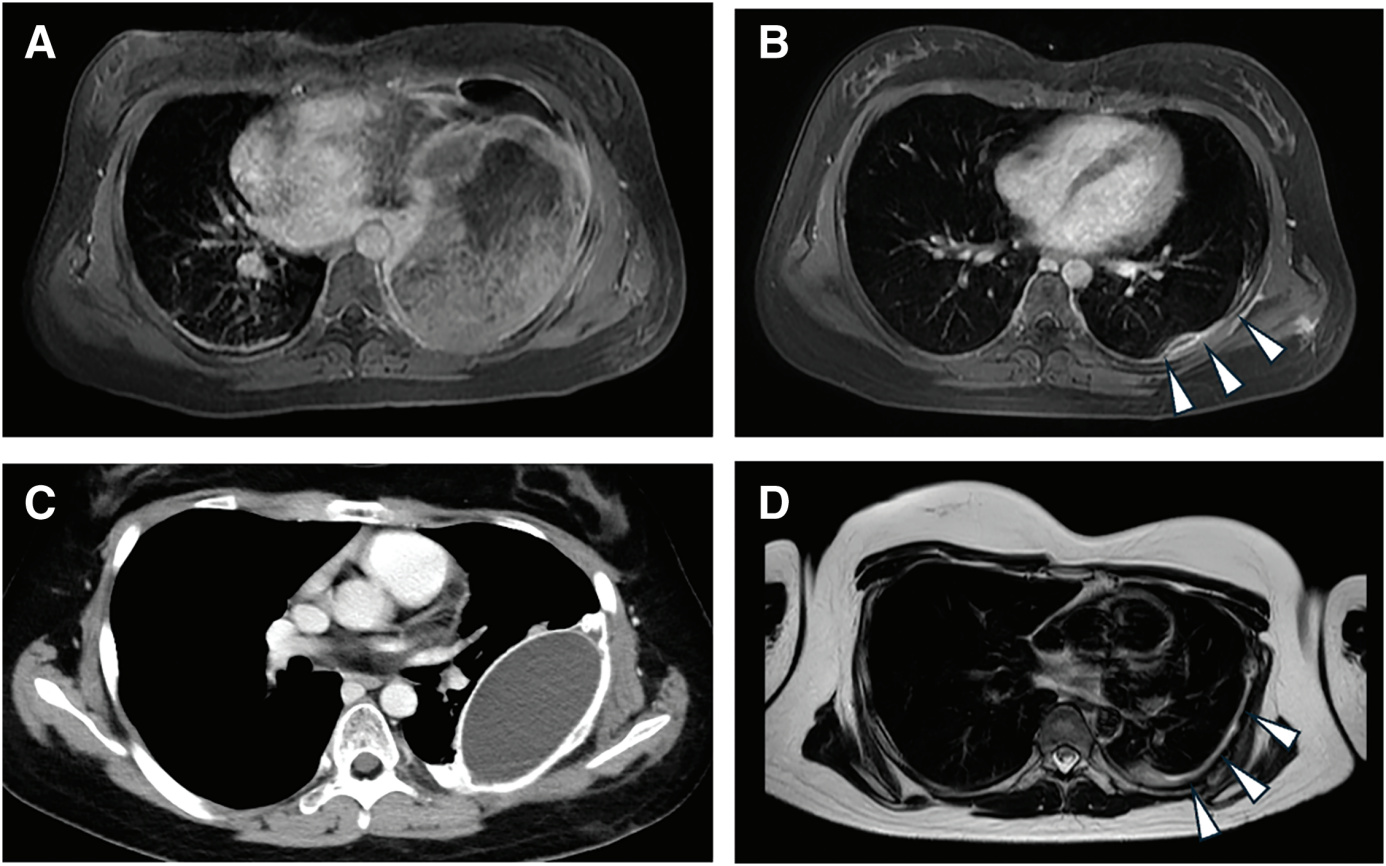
Tumor and tumor bed imaging findings (axial sections) over time. (A) MRI at diagnosis (contrast-enhanced fat-suppressed T1-weighted image). (B) MRI before definitive surgery (contrast-enhanced fat-suppressed T1-weighted image). White arrowheads indicate the residual tumor. (C) Contrast-enhanced CT after placement of the radioprotective spacer. The tissue expander–ePTFE sheet complex is depicted as an oval structure. (D) MRI 4 years after completion of treatment (T2-weighted image). White arrowheads indicate the bilayer ePTFE sheet. ePTFE, expanded polytetrafluoroethylene
